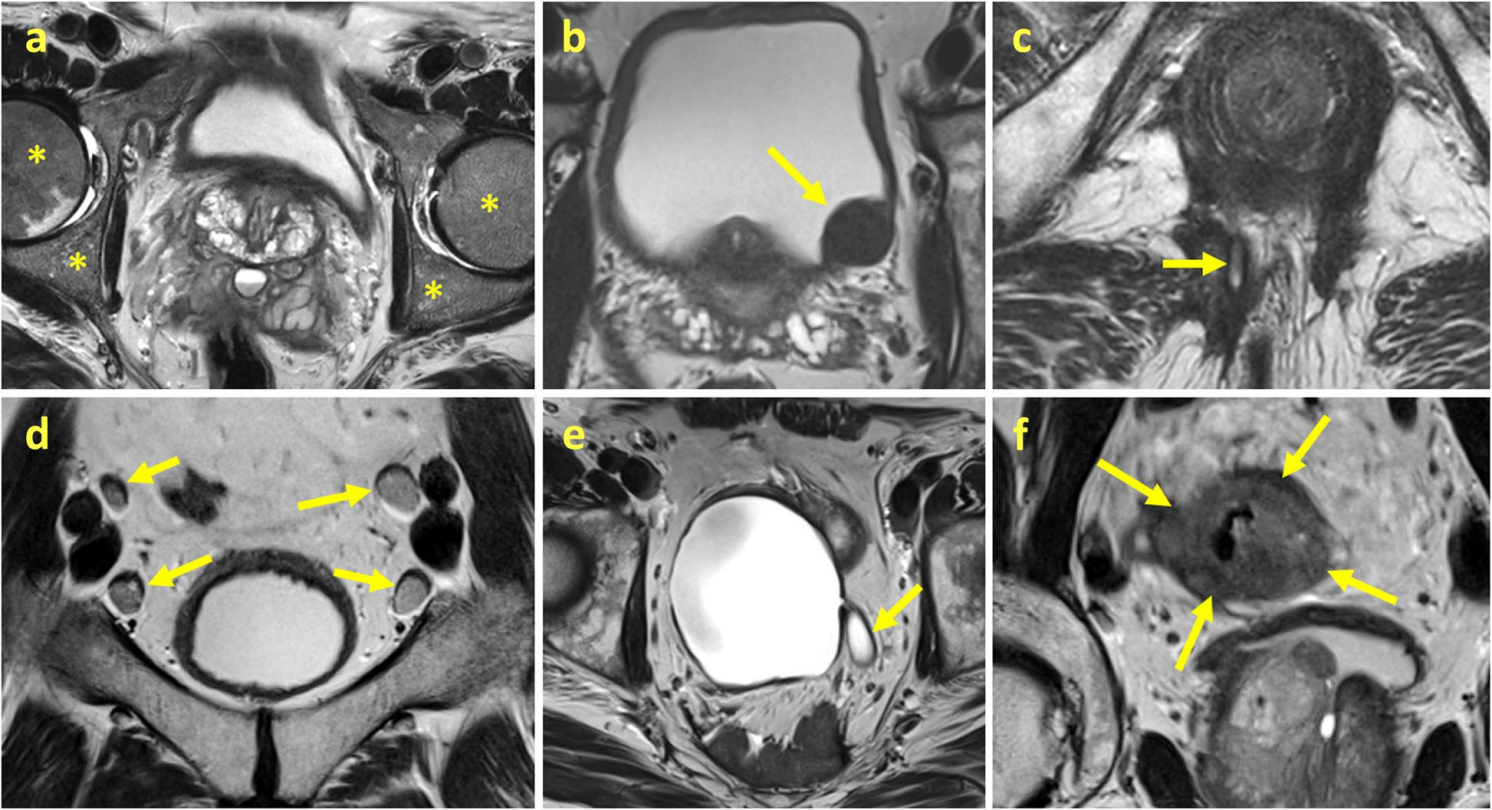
Examples of significant findings that were not included in the original report (T2-weighted fast spin echo). Diffuse bone marrow changes (a). Bladder wall tumor (b). Perianal fistula (c). Lymphadenopathy (d). Hydroureter (e). Sigmoid cancer (f)
